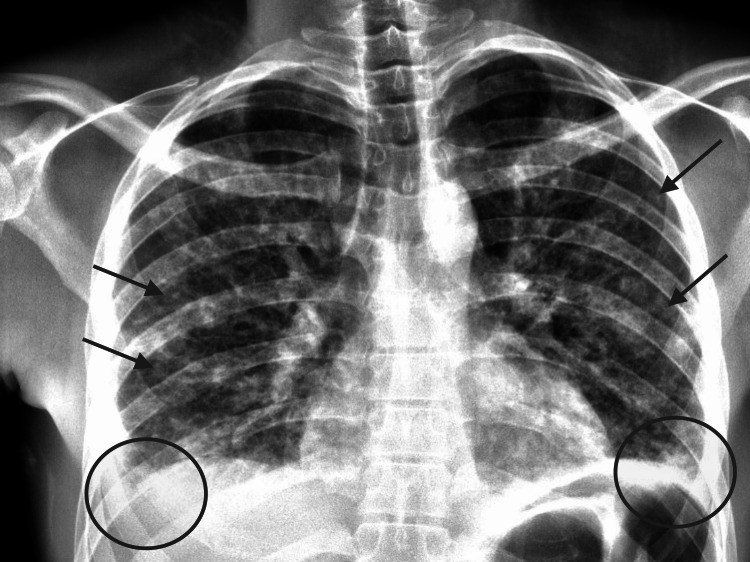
Patient’s chest X-ray at presentation Arrows indicate bilateral interstitial opacities. Circles indicate bilateral pleural effusions.
